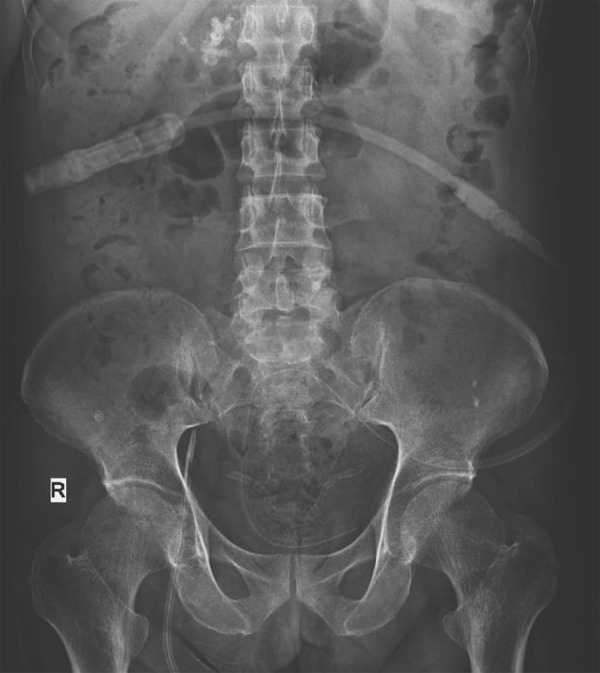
Post-CAPD catheter insertion abdominal X-ray confirming the tip position in the pelvis.
